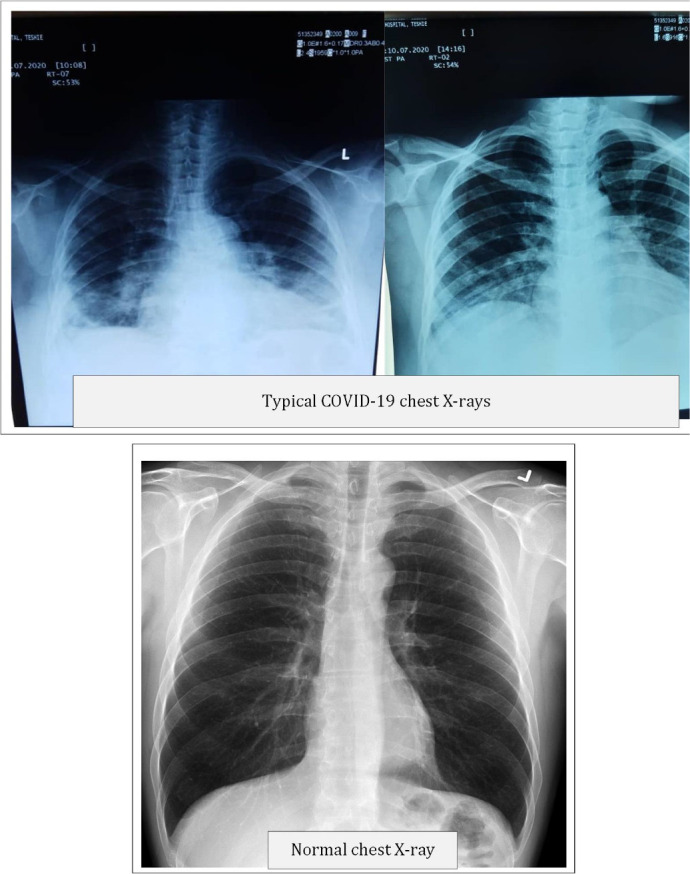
Chest radiographs in COVID-19 pneumonia compared with a normal radiograph
